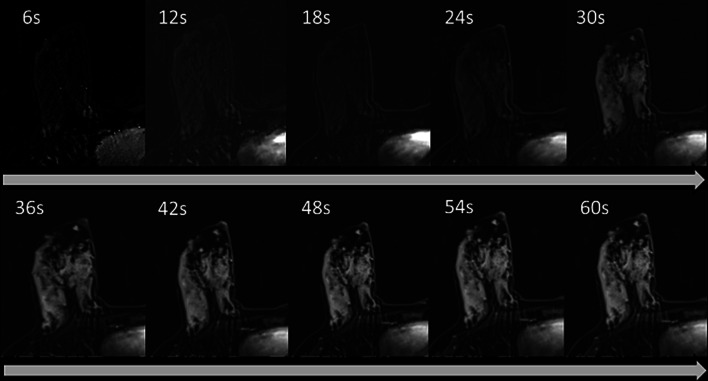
Ultrafast DCE-MRI of the lactating breast. Subtracted ultrafast DCE-MRI of the right breast of a healthy lactating patient (34 years old, lactation duration 18 months) are presented. Using grasp-vibe, a compressed-sensing technique, acquisition of ten consecutive T1-weighted images in the first minute post injection was enabled. *Note*: the prominent lactation-induced BPE (grade 3) appears only in the fifth acquired T1 image, approximately after 30 s, and further enhances in the following phases
